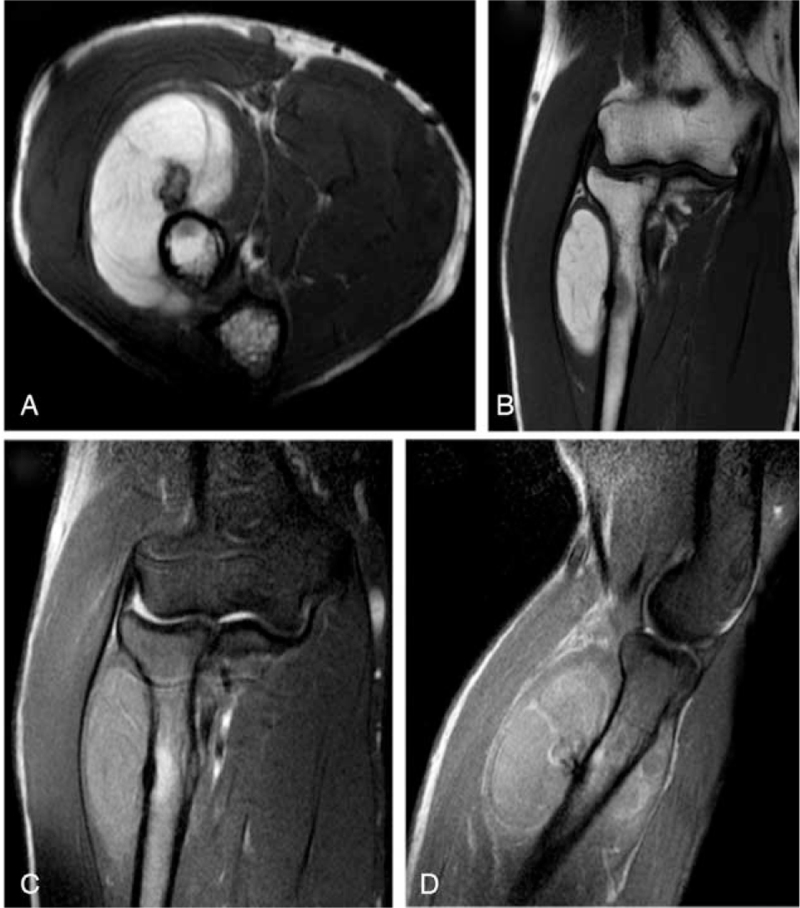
Parosteal lipoma of the proximal anterolateral forearm. Axial T1-weighted (A), coronal T1-weighted (B), coronal proton density (PD) fat saturated (C), and sagittal PD fat saturated (D) MRI of the right elbow and forearm demonstrate a lobulated parosteal lipoma with a bone protuberance projecting inside.
